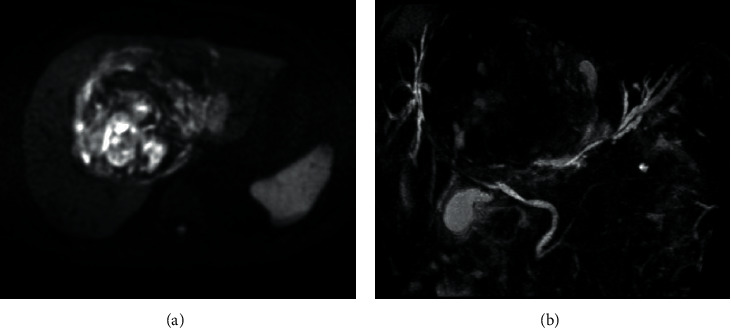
(a) Diffusion-weighted MRI images showing multiple tumors with diffusion decrease in the left lobe of the liver. (b) On MRCP, the intrahepatic bile duct was observed to be markedly compressed by bleeding in the hilum of the liver.
